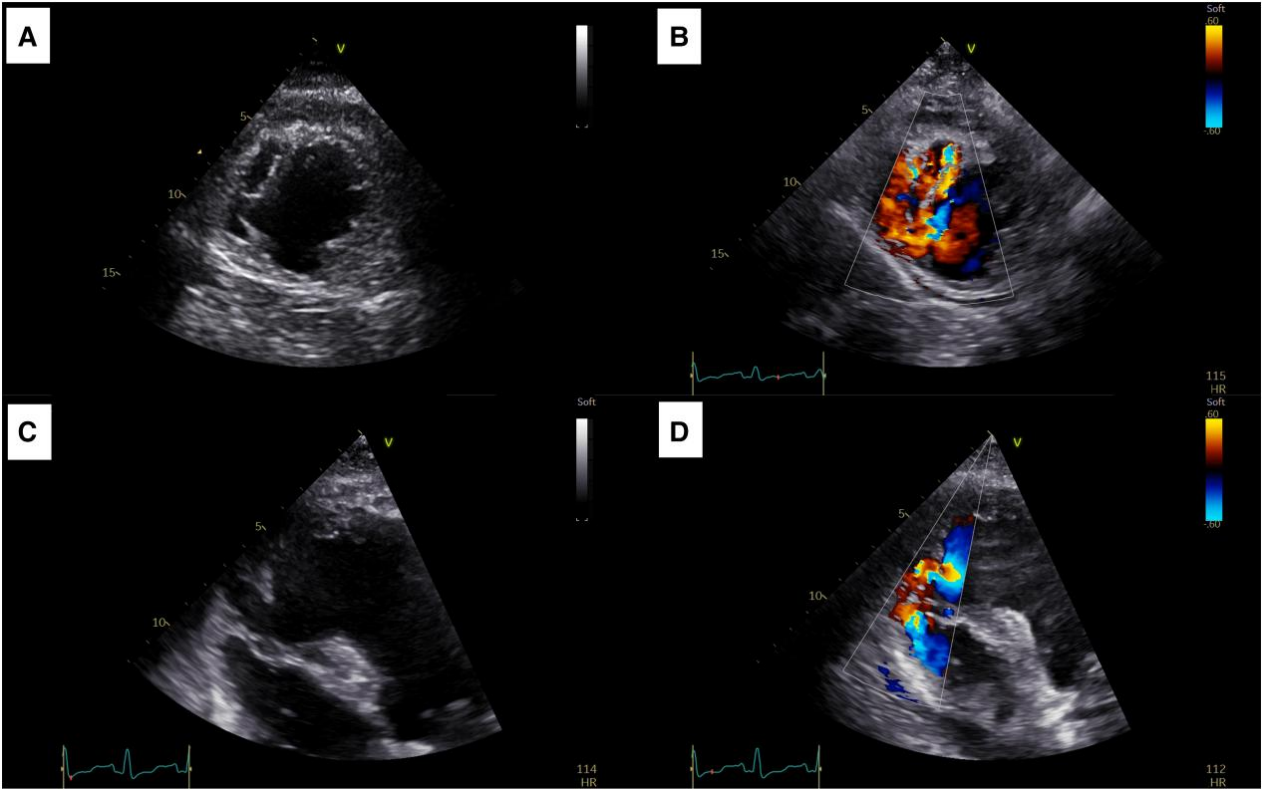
Transthoracic echocardiogram at Day 11 showing an apical ventricular septal rupture (∼19 mm) causing a left-to-right shunt (maximal gradient 60–65 mmHg). (*A*) Modified parasternal short-axis view at the apical level. (*B*) Modified parasternal short-axis view at the apical level with colour Doppler at the ventricular septal rupture location. (*C*) Modified apical four-chamber view. (*D*) Modified apical four-chamber view with colour Doppler at the ventricular septal rupture location.
